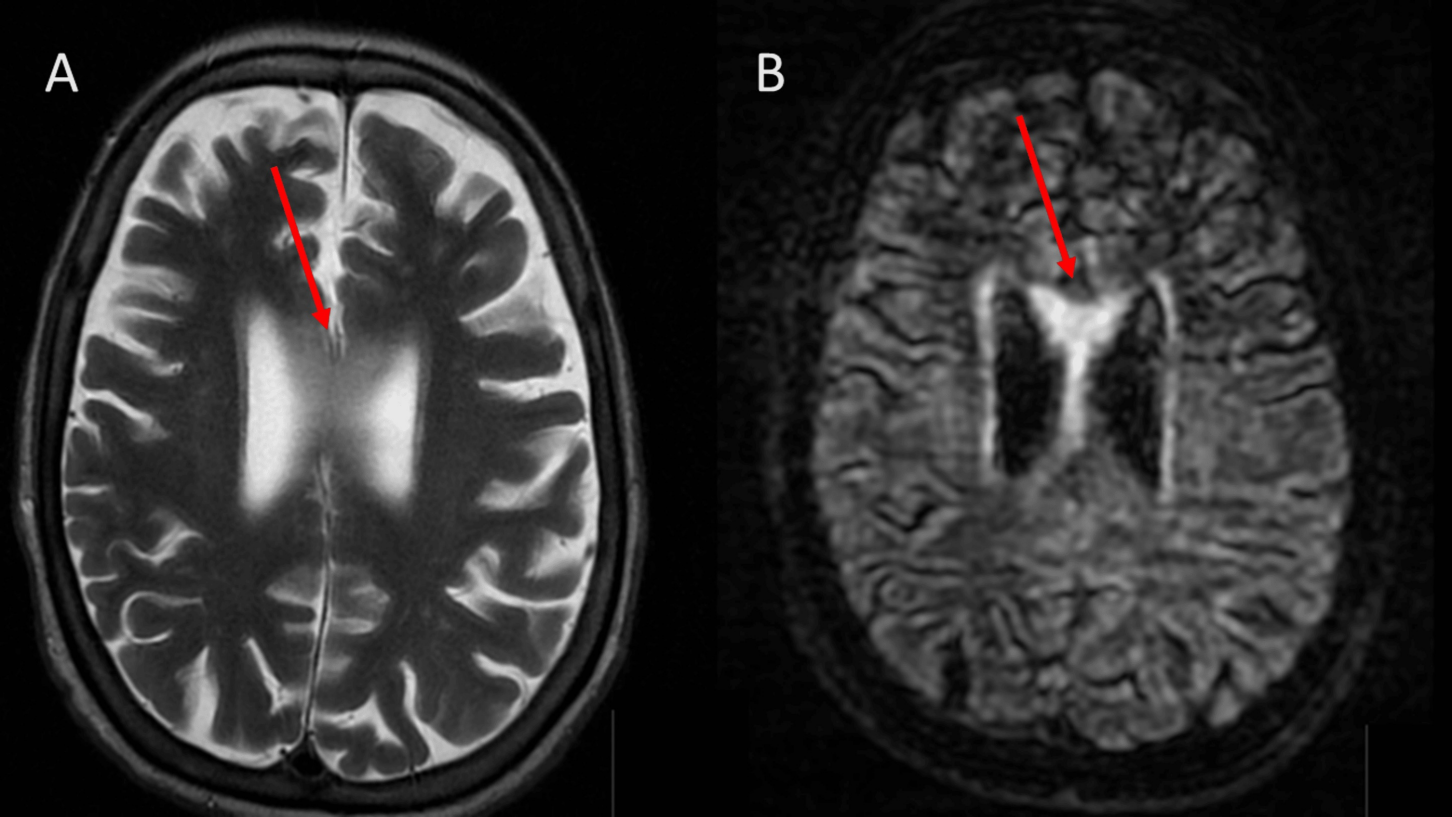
Acute demyelination due to Marchiafava-Bignami disease: T2 (A) and T2/FLAIR (B), axial MRI: Magnetic resonance imaging; FLAIR: fluid-attenuated inversion recovery Increased signal intensity affecting the anterior 2/3 of the corpus callosum, with slight associated expansion (red arrows)
